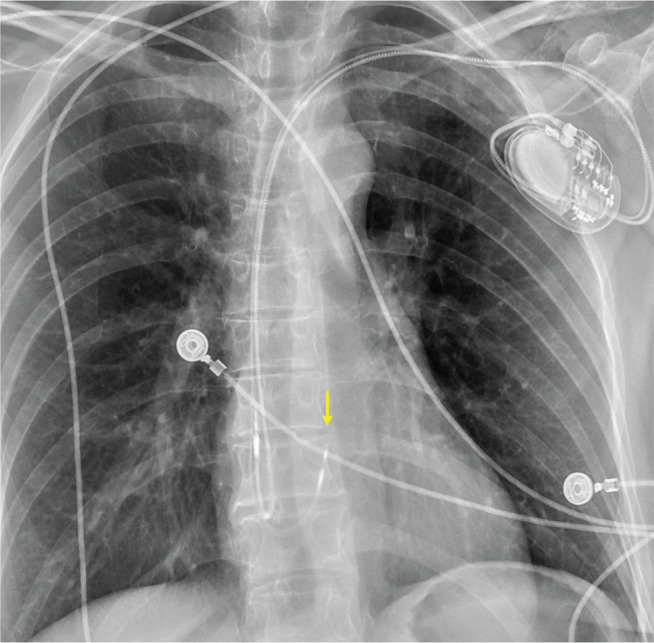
Frontal chest radiograph of a patient with a dual-chamber pacemaker that includes a His-bundle pacing lead (arrow).
